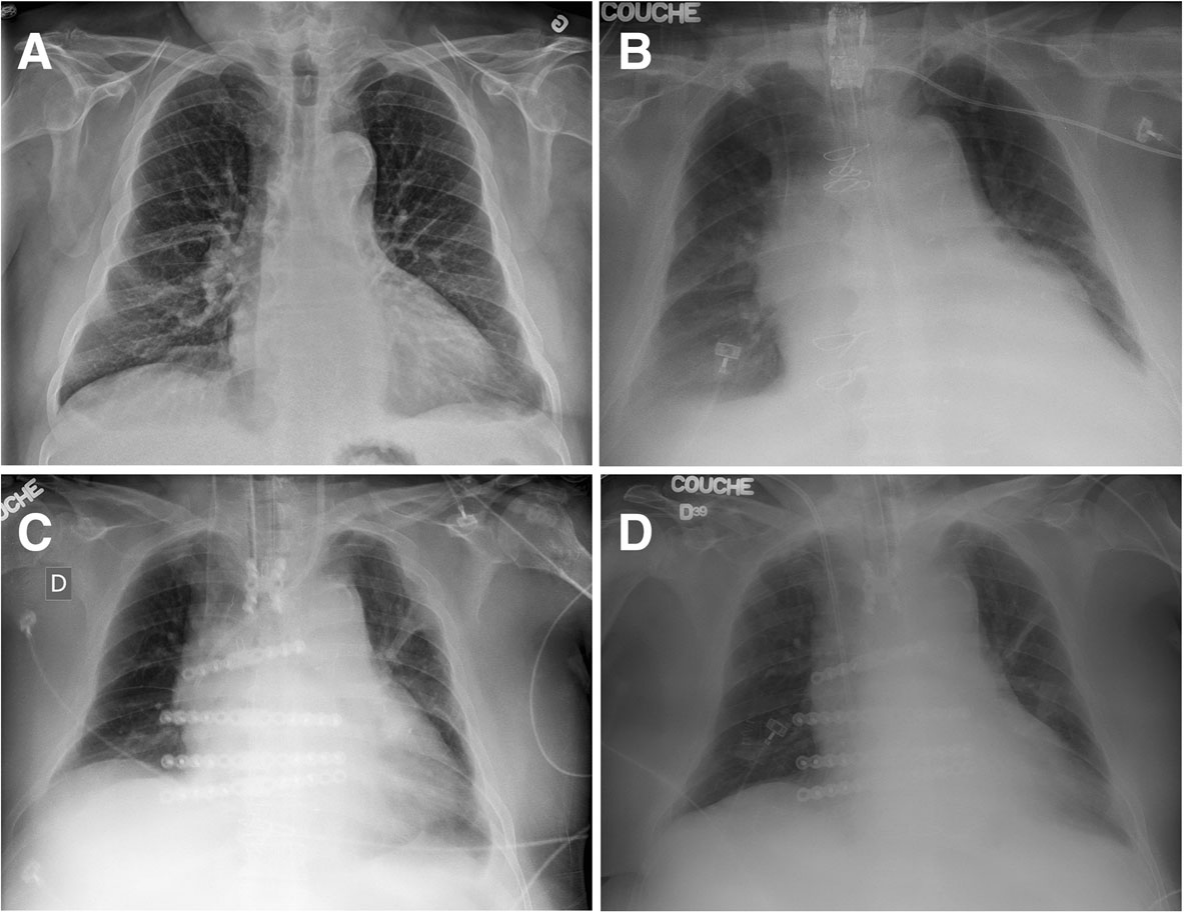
a Preoperative chest X-ray. b Postoperative chest X-ray immediately after myocardial revascularization and mitral valve replacement. c Postoperative chest X-ray immediately after the chest wall reconstruction with plates and screws. d Postoperative chest X-ray immediately after diaphragm plication
